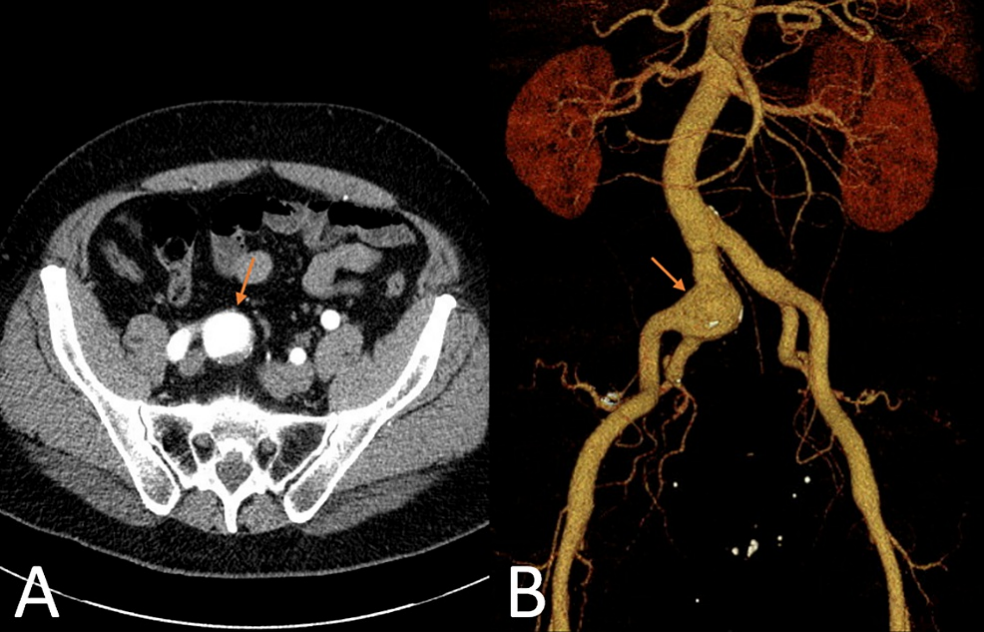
Preoperative Computed Tomography (CT) Imaging 3.7 cm fusiform aneurysm of the right common iliac artery bifurcation with slight peripheral thrombosis. The aneurysm propagates into the proximal aspect of the right internal iliac artery. (A) axial abdominal CT image. (B) Coronal abdominal CT image with three-dimensional (3D) reconstruction. Orange arrow: common iliac artery aneurysm.
